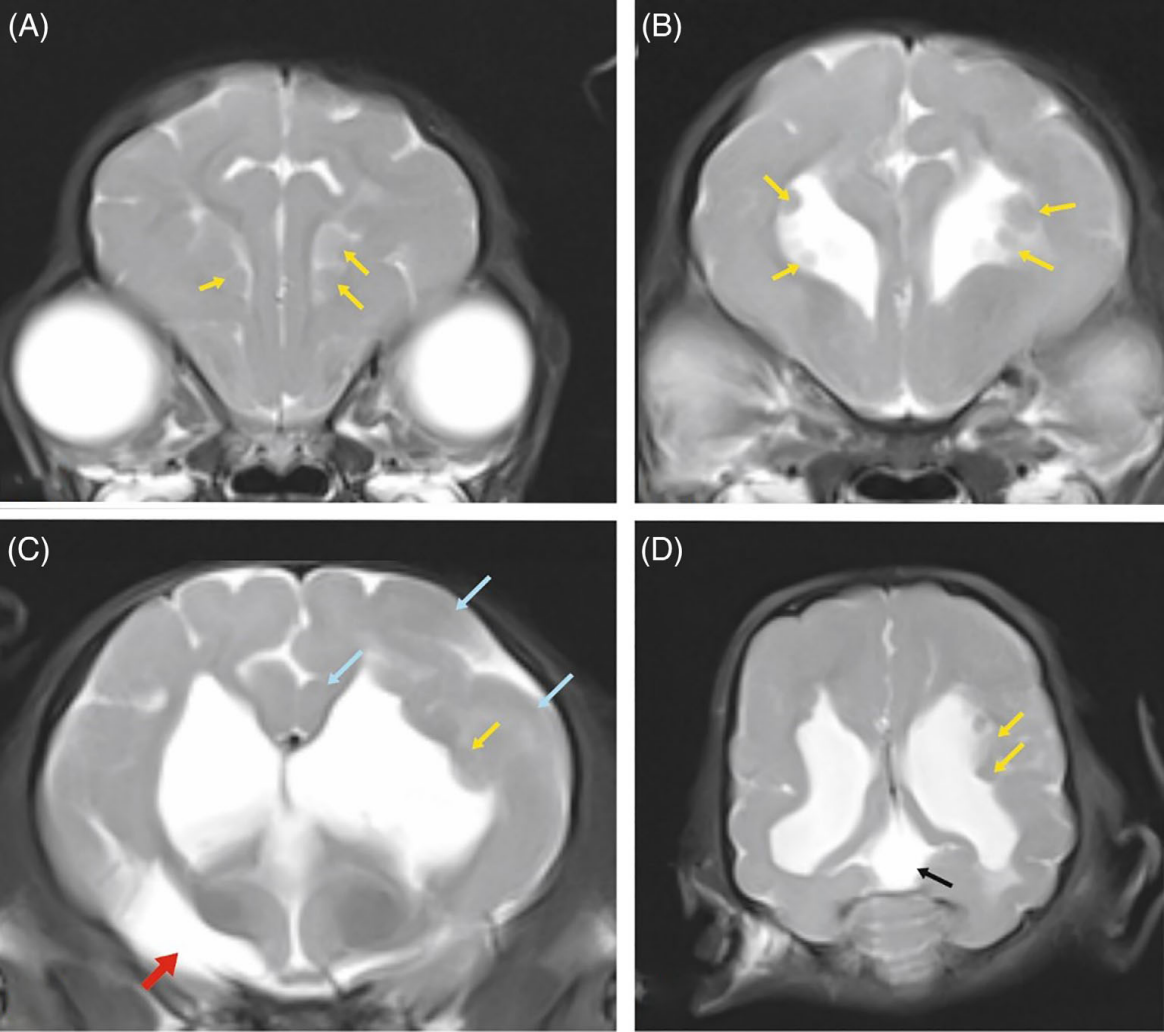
A, B, C, Transversal and D, dorsal T2‐weighted magnetic resonance images of a Chihuahua. Single round to ovoid nodules (yellow arrows) line the inner surface of the lateral cerebral ventricles on both sides. The nodules have the same signal intensity as the overlying gray matter. The gyrification pattern is irregular with smaller and larger gyri compared with the other hemisphere (blue arrow). There is bilateral symmetrical ventriculomegaly of the lateral cerebral ventricles and a supracollicular fluid accumulation (black arrow). The corpus callosum is thin, C. There is a large irregular parenchymal defect in the right ventral thalamus filled with cerebrospinal fluid (red arrow)
